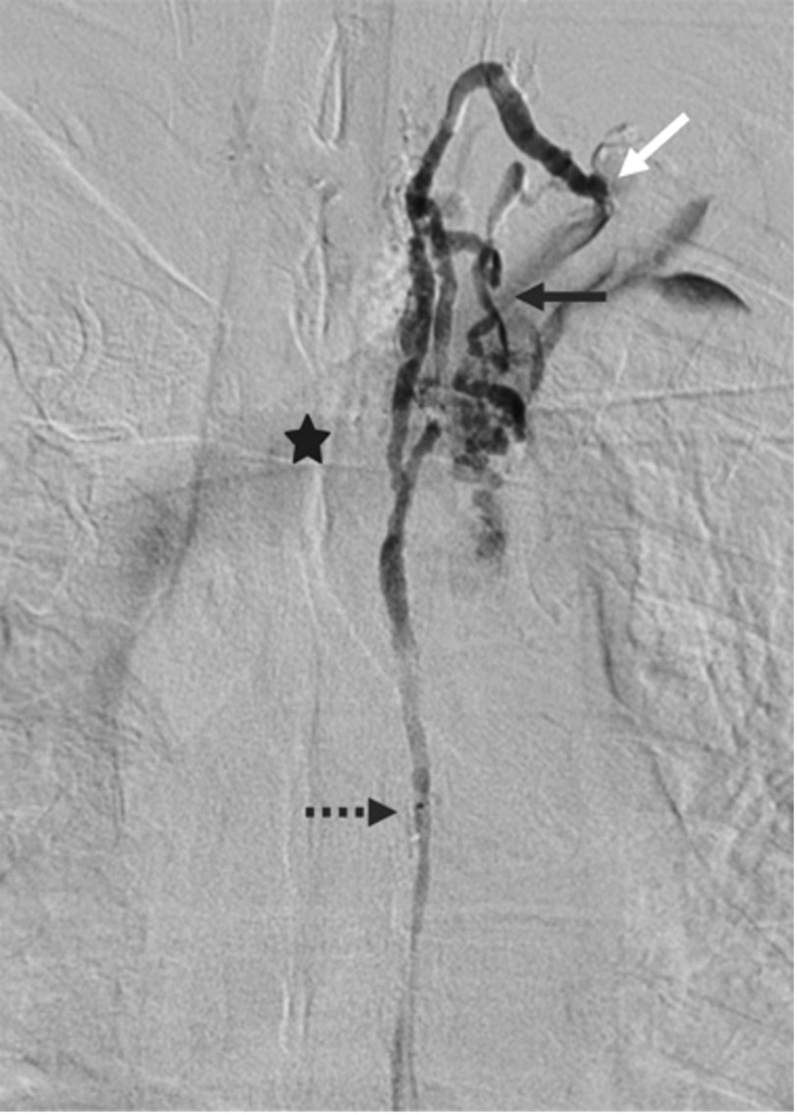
Catheter Lymphangiogram Digital subtraction lymphangiogram via a microcatheter in the midthoracic duct at the level of the carina (dashed arrow). Iodinated contrast flows through the lymphovenous junction (white arrow) into the brachiocephalic vein (black star). The source of the leak is reflux in the left anterior mediastinal branch (black arrow). Contrast injection from catheter-based lymphangiography demonstrated a patent thoracic duct with reflux into a left anterior mediastinal branch, extending into the mediastinum and corresponding to the suspected pericardial leak seen on magnetic resonance imaging.
